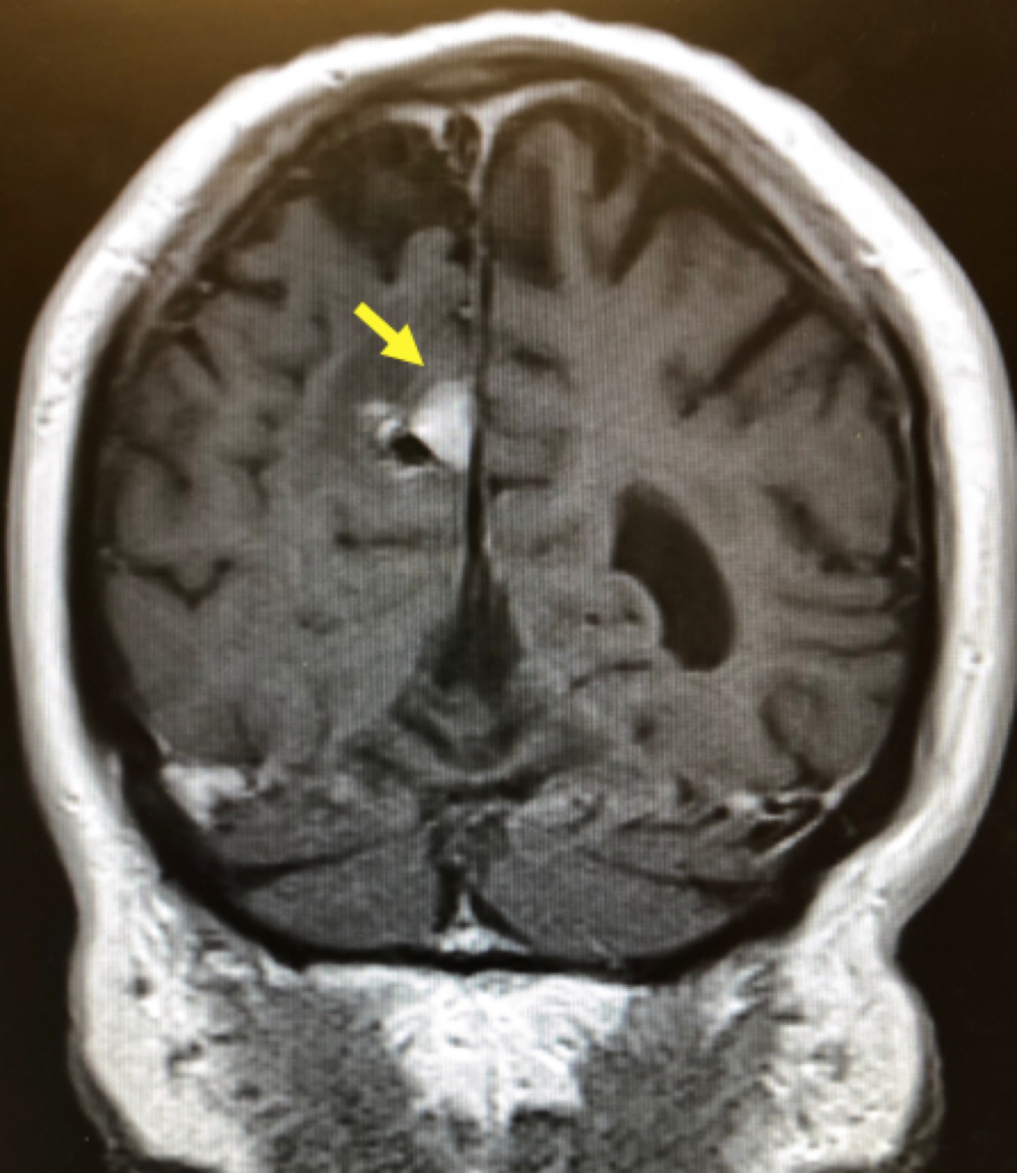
MRI brain with and without contrast demonstrating an enhancing lesion in the right frontal cortex 5 mm x 7 mm in diameter MRI: magnetic resonance imaging
